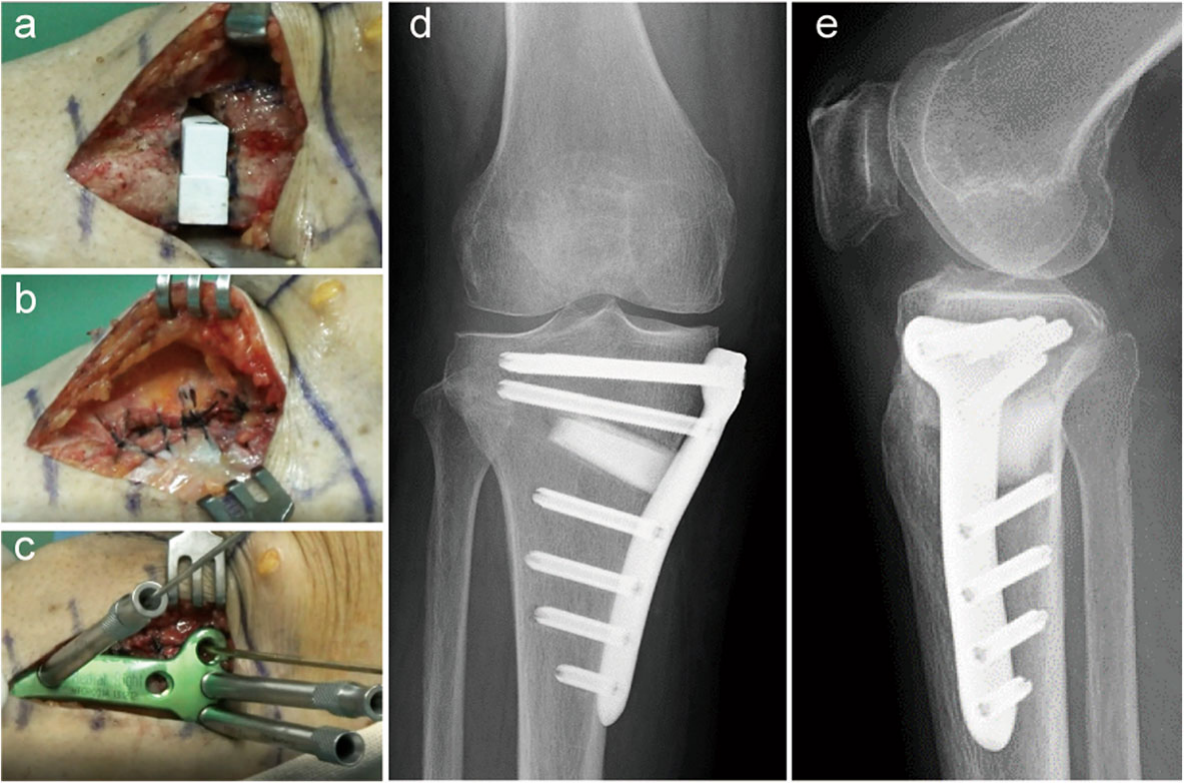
a Equivalent to the size of the opening, the wedged β-tricalcium phosphate spacers (Osferion60, Olympus Terumo Biomaterials) are inserted into the osteotomy site to fit into the medial cortical bone edge. b sMCL, pes anserinus, and periosteum are repaired. c The locking plate (TriS Medial HTO Plate System, Olympus Terumo Biomaterials) is fixed on the medial aspect of the proximal tibia. Postoperative anteroposterior (d) and lateral (e) radiographs of the right knee show a low-profile locking plate (TriS Medial HTO Plate System, Olympus Terumo Biomaterials) fixed in place with eight locking screws
